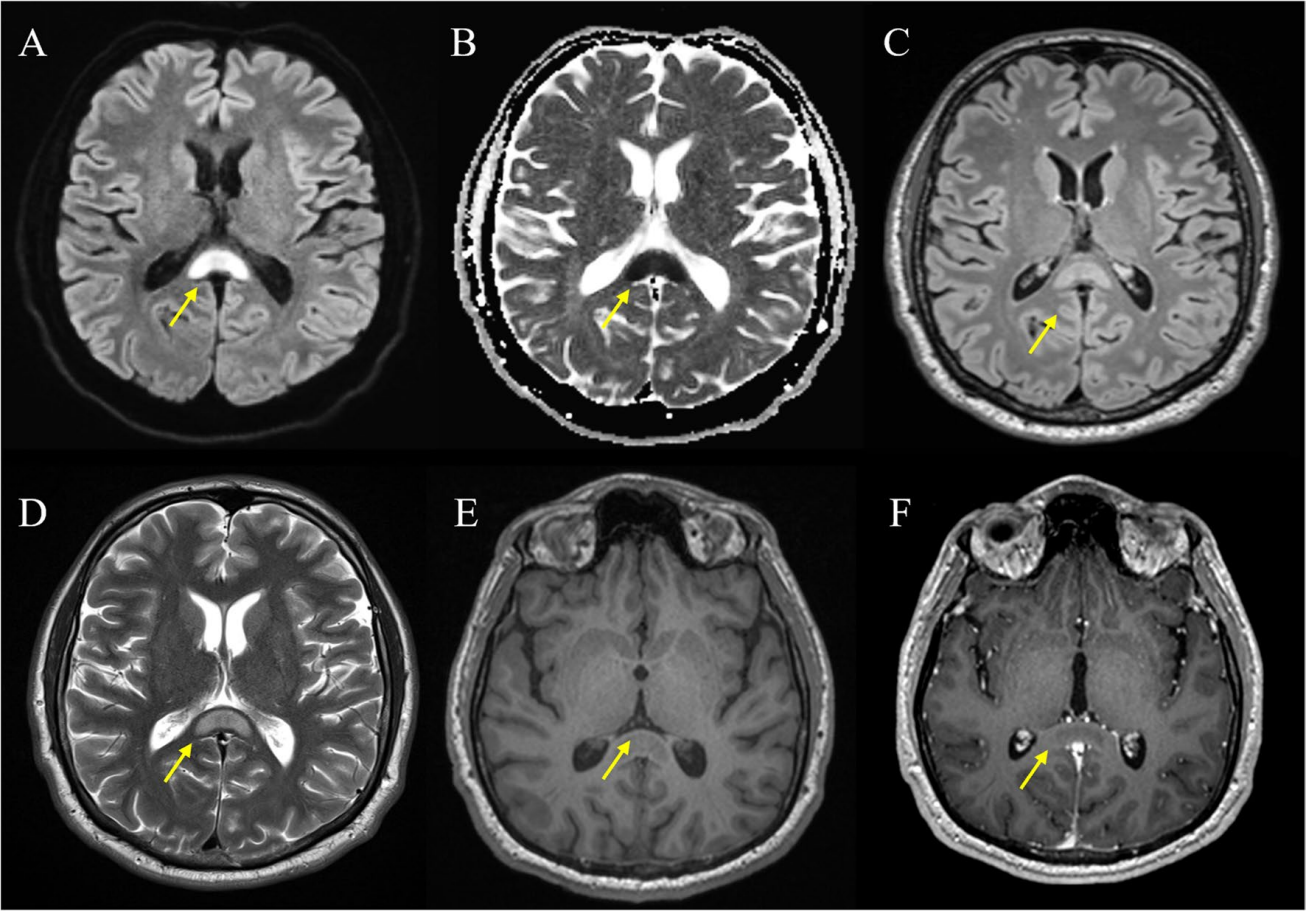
Typical structural MRI findings in cytotoxic lesions of the corpus callosum (CLOCC). Pictorial magnetic resonance imaging (MRI) examples of a 38-year-old patient who presents with a typical cytotoxic lesion of the corpus callosum (CLOCC) after head trauma. A DWI B0, oval hyperintensity throughout the splenium and into the adjacent hemispheres (“boomerang sign”). B ADC hypointensity due to restricted diffusion. C T2w-FLAIR, high signal. D T2 slightly hyperintense. E T1 native, pre-gadolinium uptake, shows a slight hypointensity in the region. F T1 post-gadolinium uptake, no enhancement. Abbreviations. DWI, diffusion-weighted imaging; FLAIR, fluid-attenuated inversion recovery
